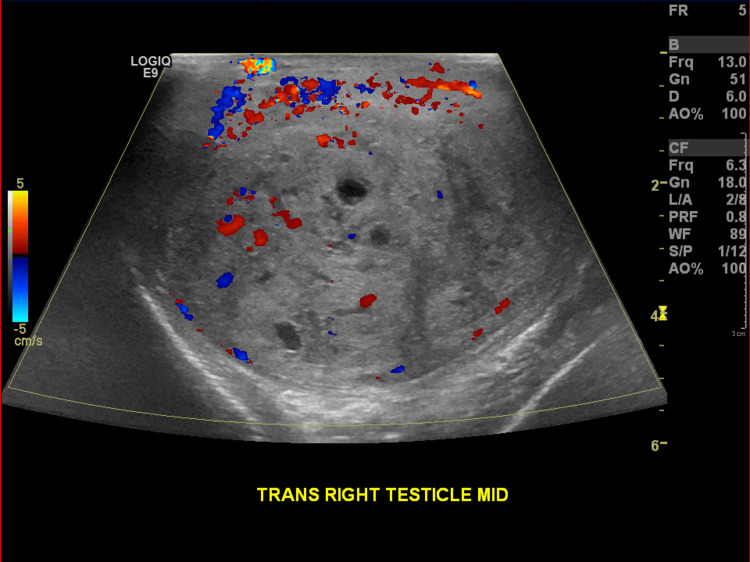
Sonographic image of the scrotum demonstrating a large heterogenous mass replacing the right testicle The mass demonstrates internal vascularity, calcifications, and cystic spaces.
